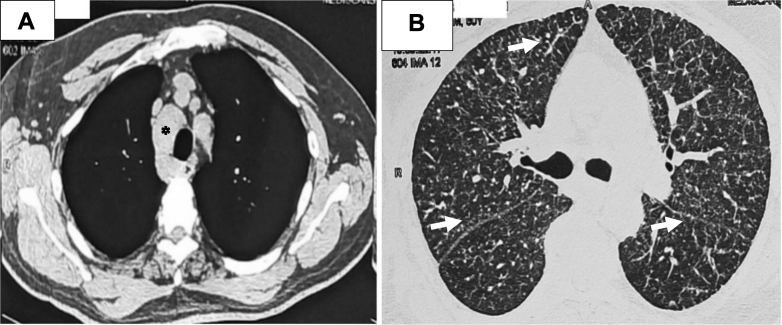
A, Axial section of a chest CT scan with IV contrast showing mediastinal lymphadenopathy (right paratracheal node denoted by black asterisk). B, Parenchymal cuts of an axial section of a chest CT scan showing diffuse perilymphatic nodules (white arrows).
